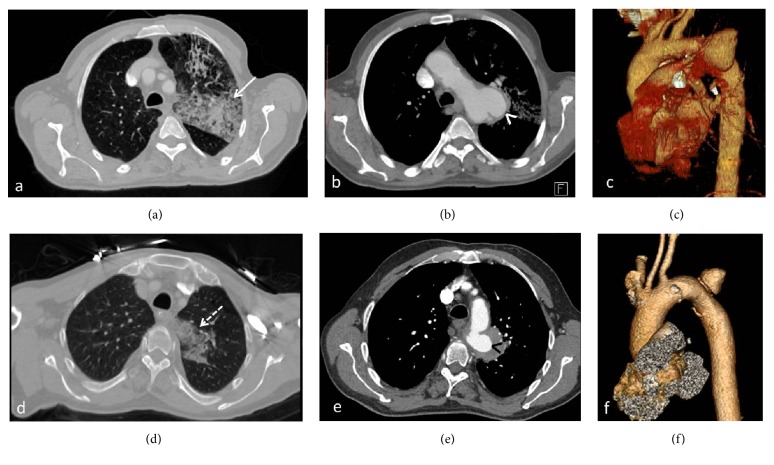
Case 1 (a–c). Case 2 (d–f). Contrast-enhanced CT and volume rendering technique (VRT) images revealed parenchymal hemorrhages in the left upper lungs (white arrow in (a), dashed arrow in (d)) and saccular aneurysms located proximal to the descending thoracic aorta (white arrow head in (b) and black arrow head in (e)).
